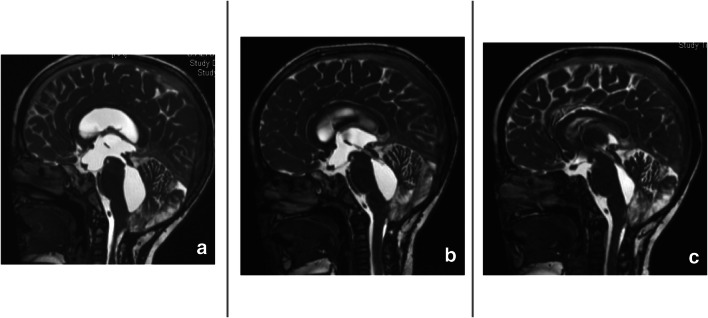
MR images demonstrating TFV in a child after resection of medulloblastoma. Patient presented with fatigue, bilateral abducens paresis, and ataxia denoting VP shunt dysfunction as well as TFV. For the purpose of the planned intrathecal chemotherapy, aqueductoplasty with stenting was done to ensure application of the chemotherapy to the local tumor bed in the fourth ventricle. a Mid-sagittal CISS MR image showing the TFV. b, c Mid-sagittal CISS MR images showing the stent in place immediate postoperative and 1.5 years later respectively
